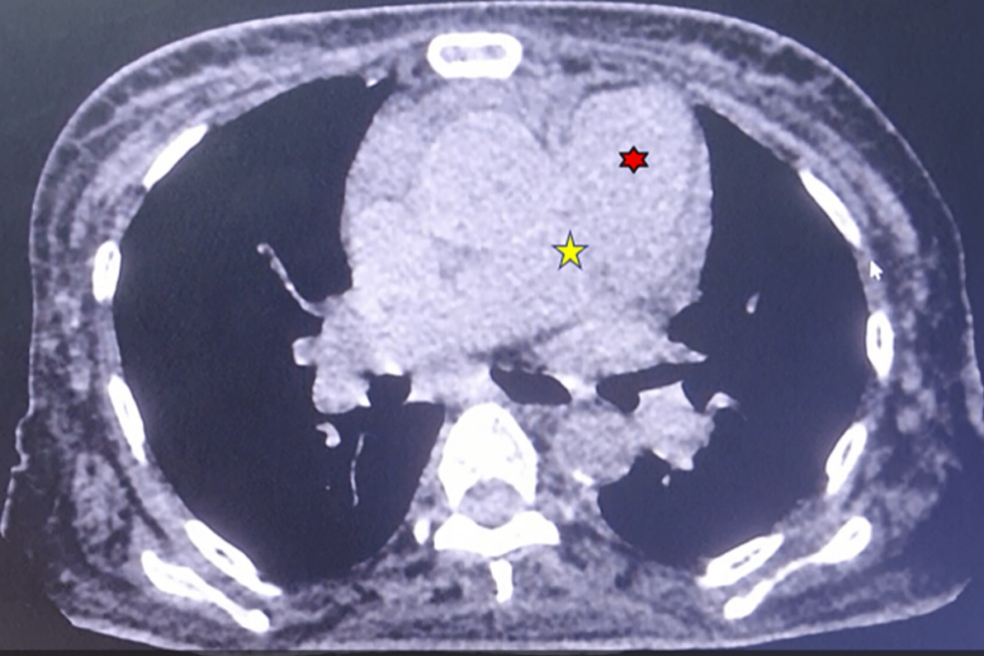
CT scan of the chest showing a dilated main pulmonary trunk (red) and right branch of the pulmonary artery (yellow)
